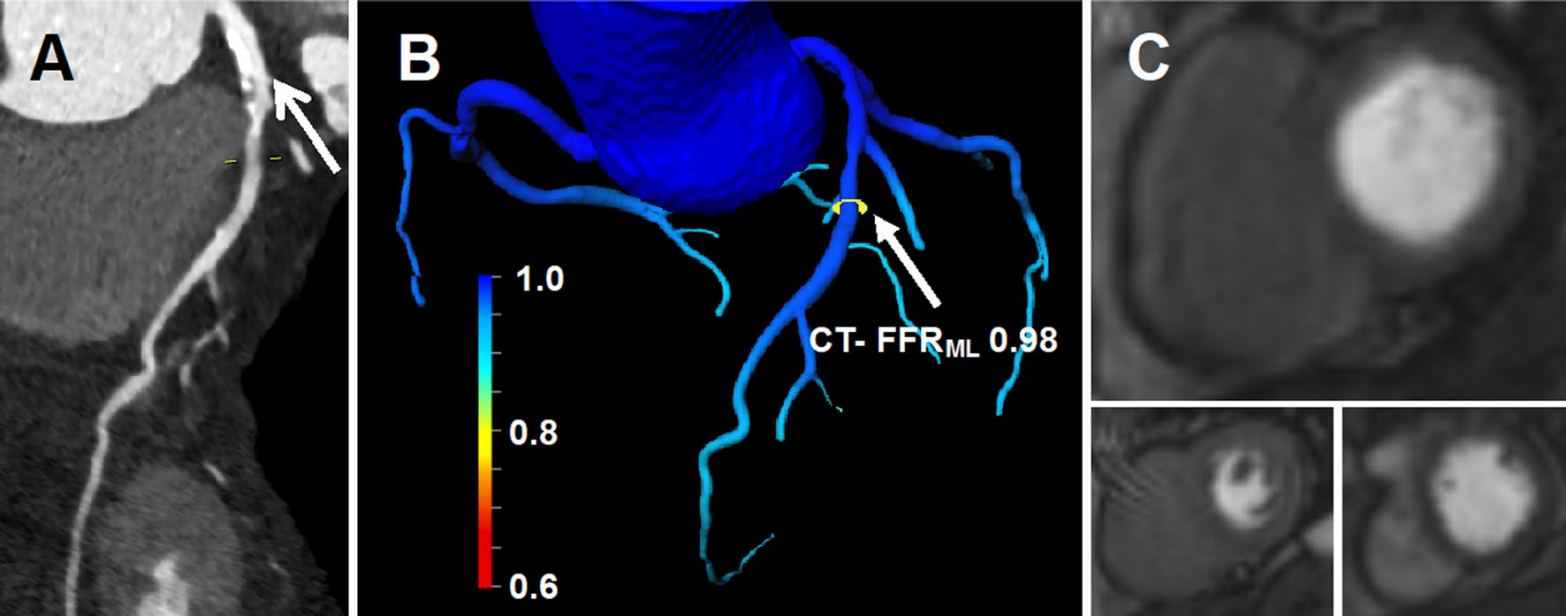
80-year-old male patient with pre-test probability of CAD of 37% (CAD consortium (%)- basic model). a cCTA demonstrates a high-risk plaque in the main stem of the left coronary artery and in the proximal left anterior descending coronary artery (arrow). b CT-FFR_ML_ software provides a color-coded 3-dimensional mesh of the coronary tree and calculated a value of 0.98 in an area distal of the stenosis (arrow). c Stress perfusion CMR in three short axis slices shows no perfusion deficit and thus no myocardial ischemia. CAD = coronary artery disease; cCTA = coronary CT angiography; CT-FFR_ML_ = Fractional flow reserve derived from coronary computed tomography angiography based on machine learning algorithm; CMR = cardiovascular magnetic resonance imaging
